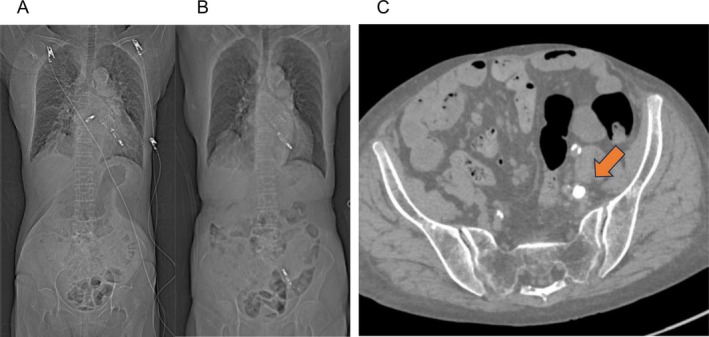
Imaging findings of atrial leadless pacemaker embolization. (A) CT scan confirming the atrial leadless pacemaker in the right atrium. (B) CT showing migration of the device into the left lower extremity. (C) Axial non‐contrast CT demonstrating the atrial leadless pacemaker lodged in the left common iliac vein (orange arrow).
